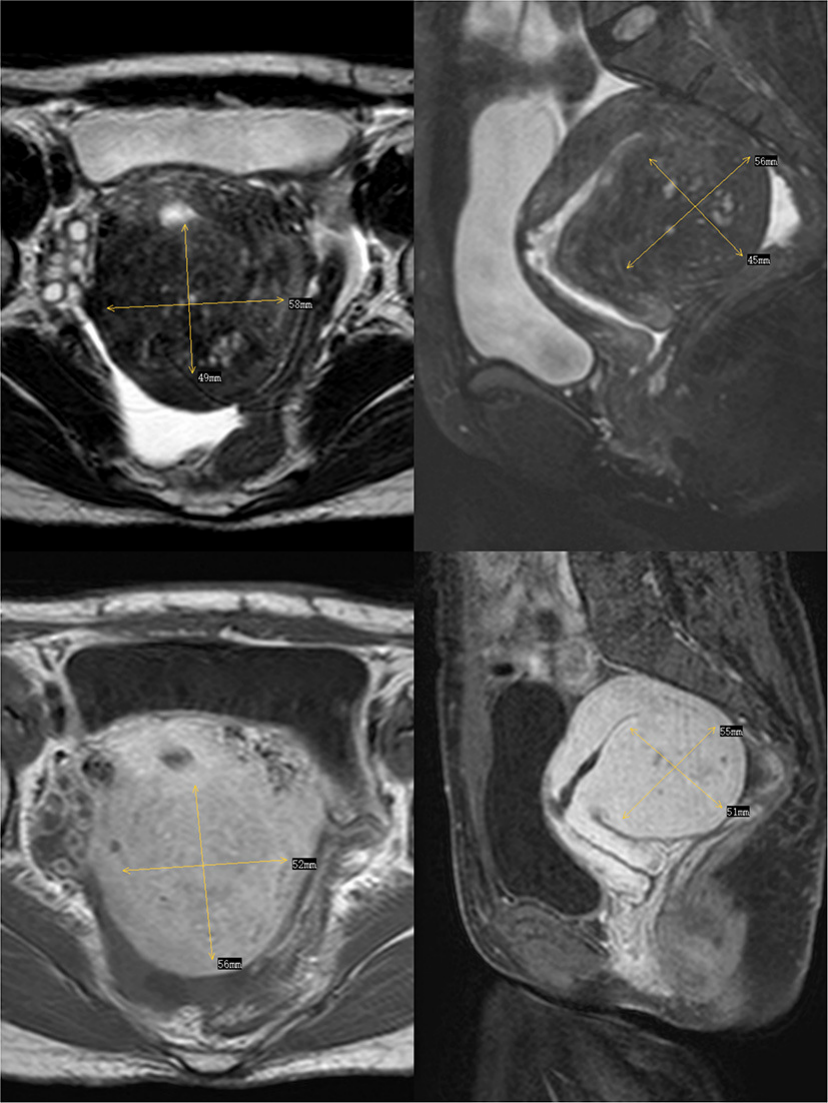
The above two images are T2-weighted images of the transverse and longitudinal sections of the uterus respectively, and the following two images are enhanced images of T1.
